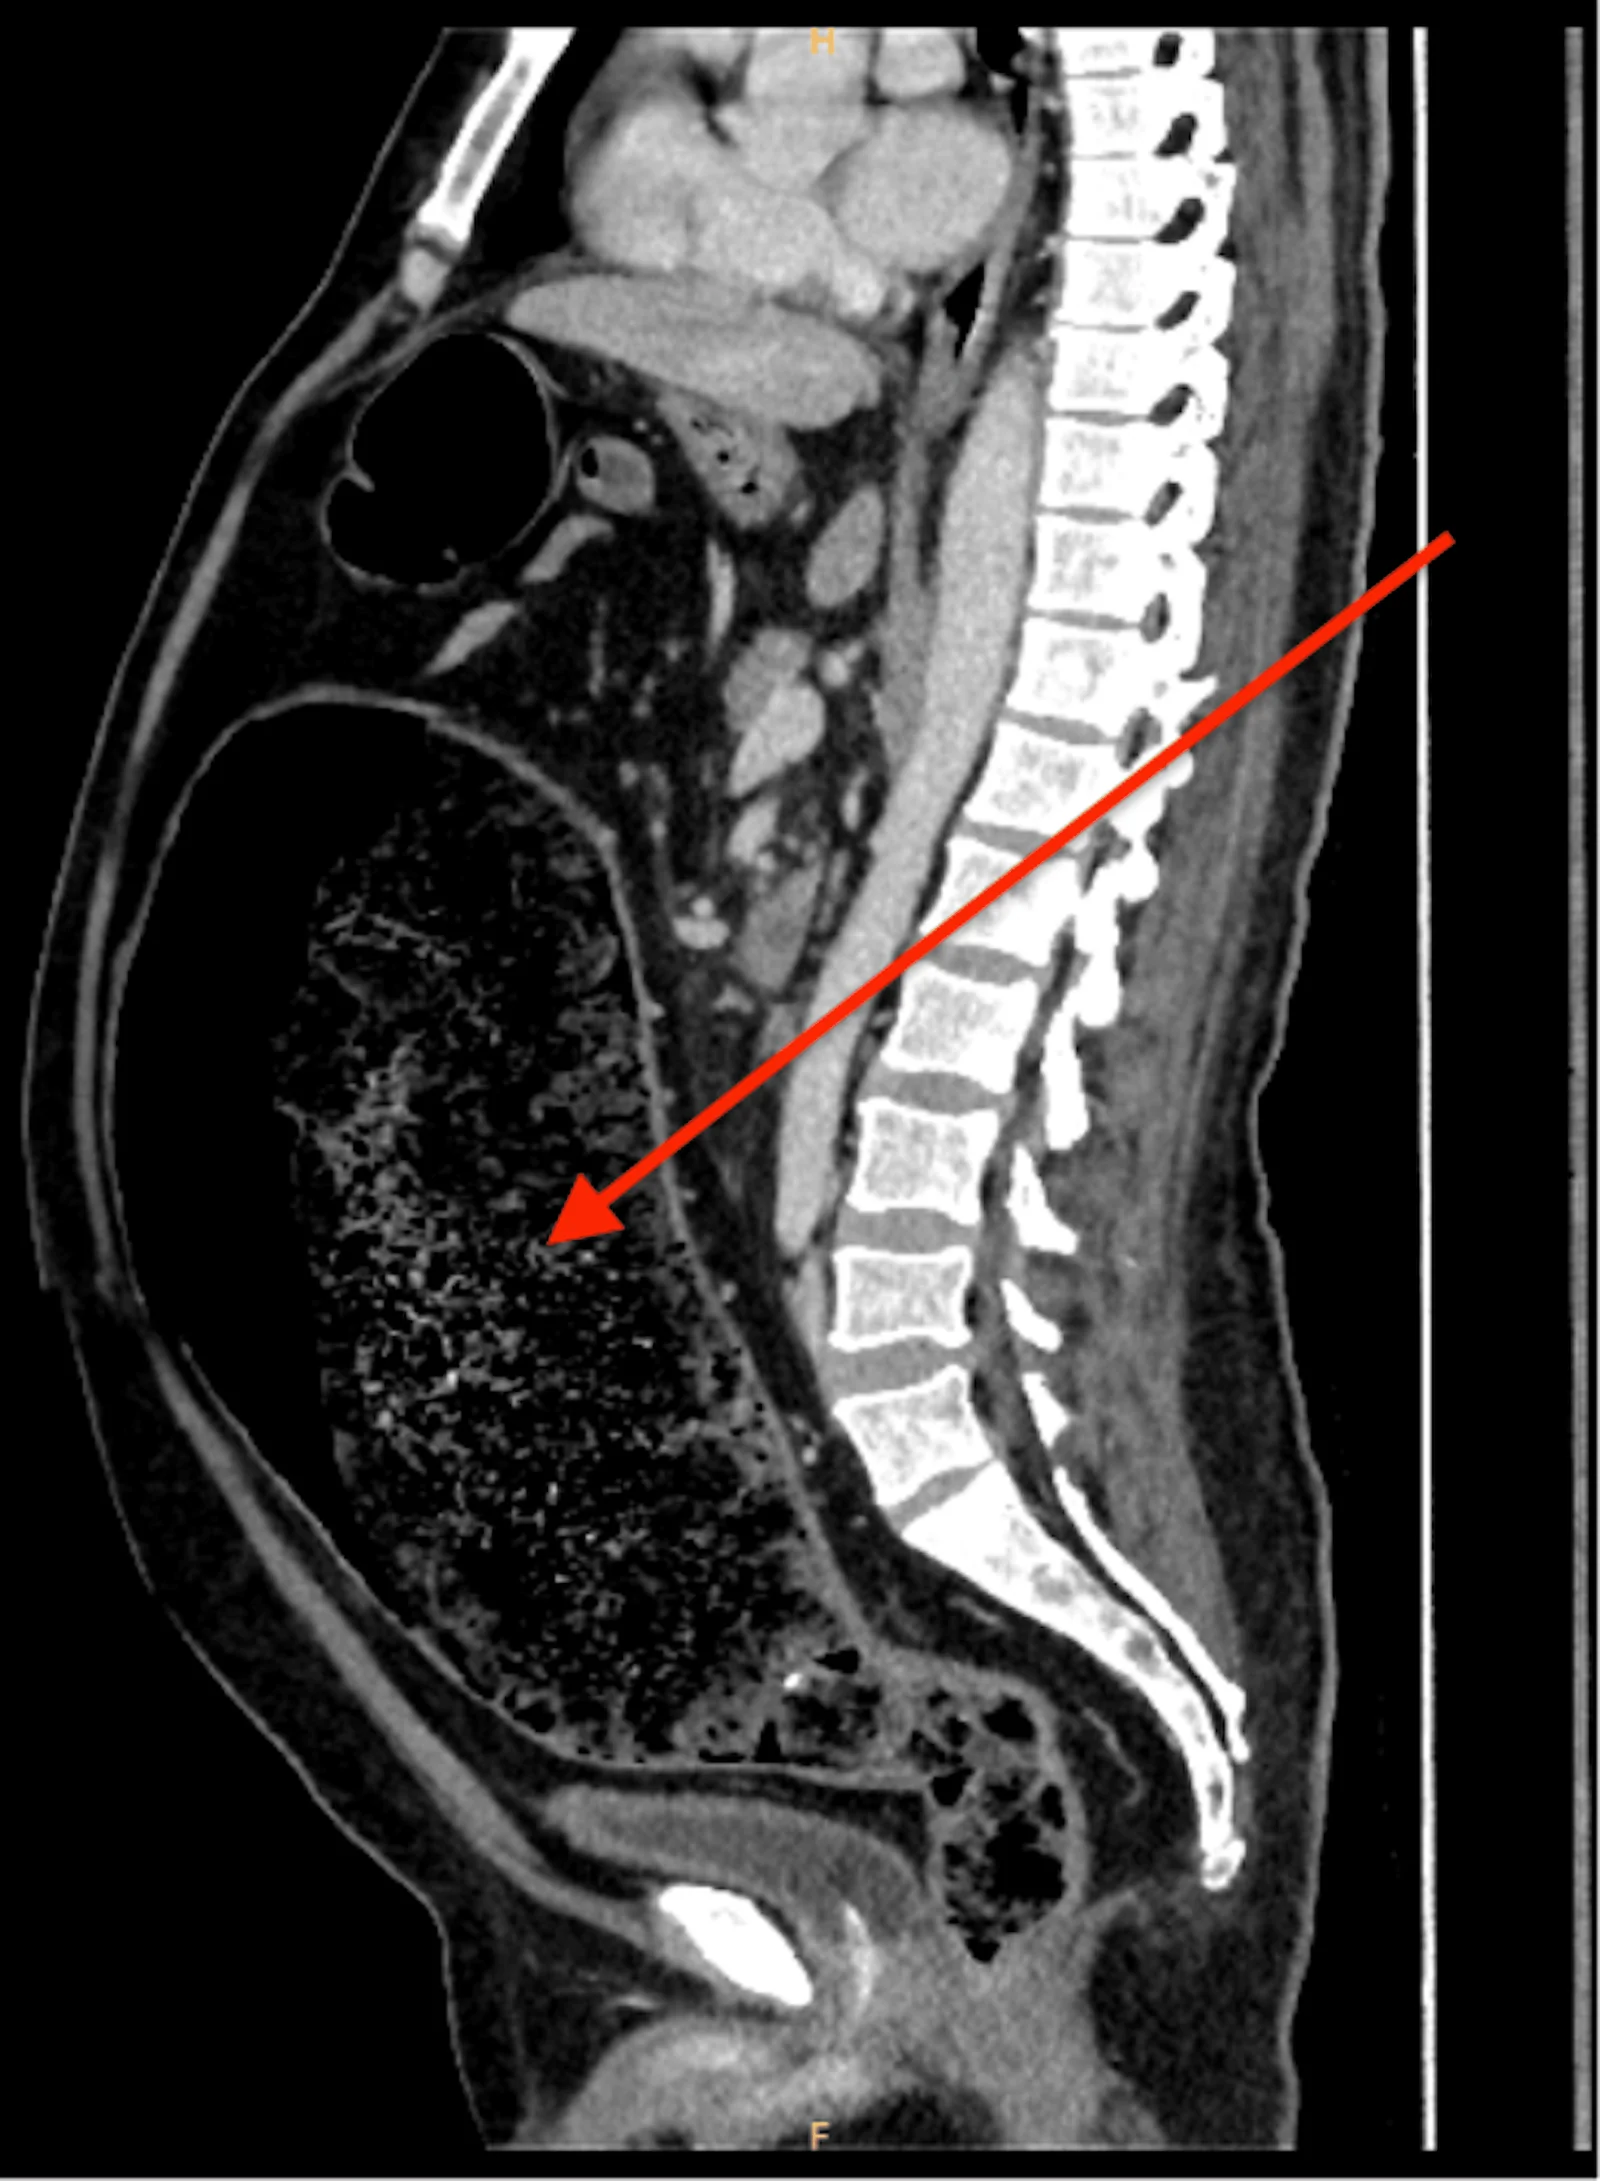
Computed tomography (CT) abdomen - sagittal section Sagittal contrast-enhanced CT abdomen showing an extensive rectosigmoid fecaloma (red arrow) occupying the dilated colon with associated diffuse colonic dilatation and ileus, consistent with severe fecal impaction.
